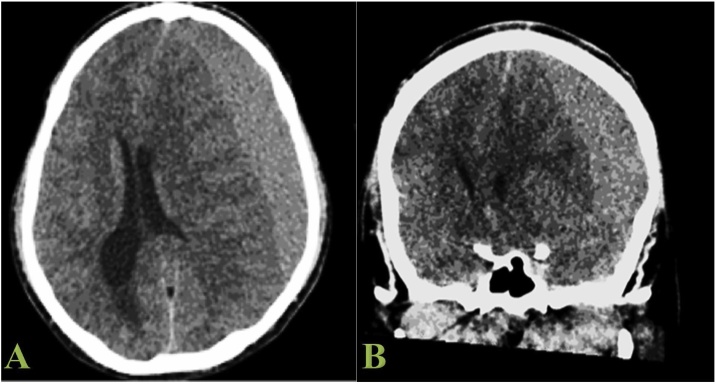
Brain CT coronal (1A) and axial (1B) views showed left sub-acute SDH.
